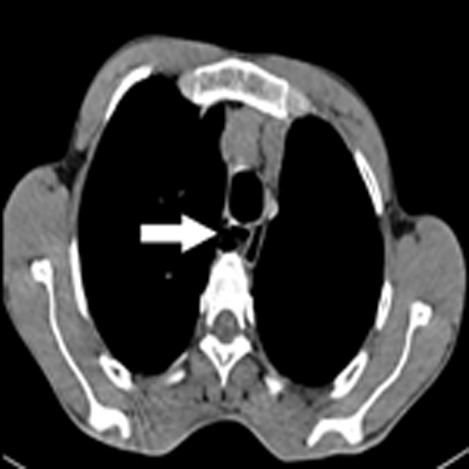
computed tomography of the chest with a white arrow showing multiple tracheal diverticula
